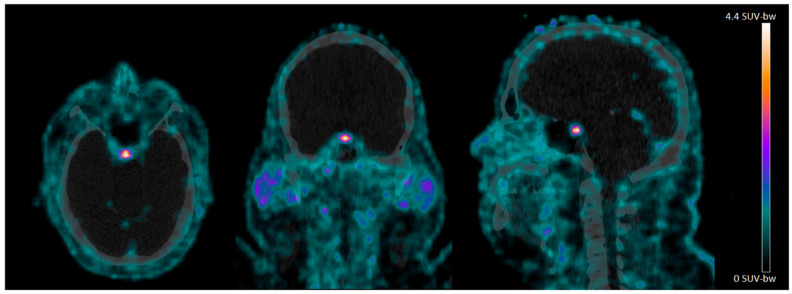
^68^Ga-NODAGA-exendin-4 PET/CT scans of the head acquired 60 min after injection in an obese subject. Note the intense uptake in the pituitary.
